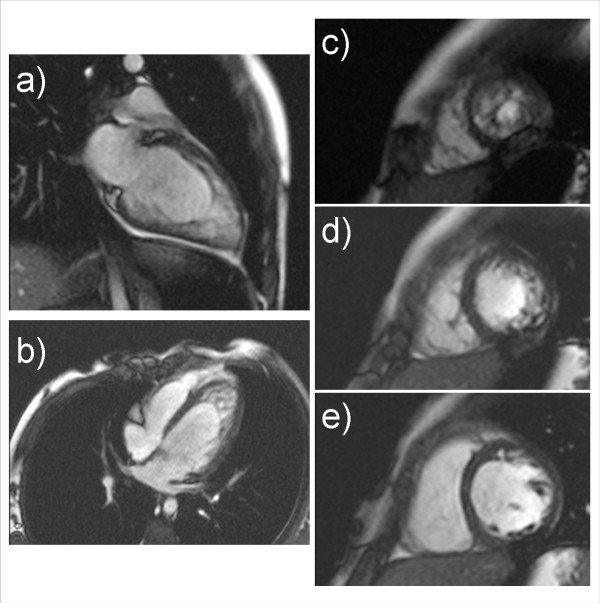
A five years old female with EF 41.5%. Steady-state free precession magnetic resonance imaging in a Long Axis (a), four-chamber (b) and short-axis (c, d, e) views demonstrate extensive trabeculations of the LV wall in the basal (e), mid (d) and apical segments (c).
